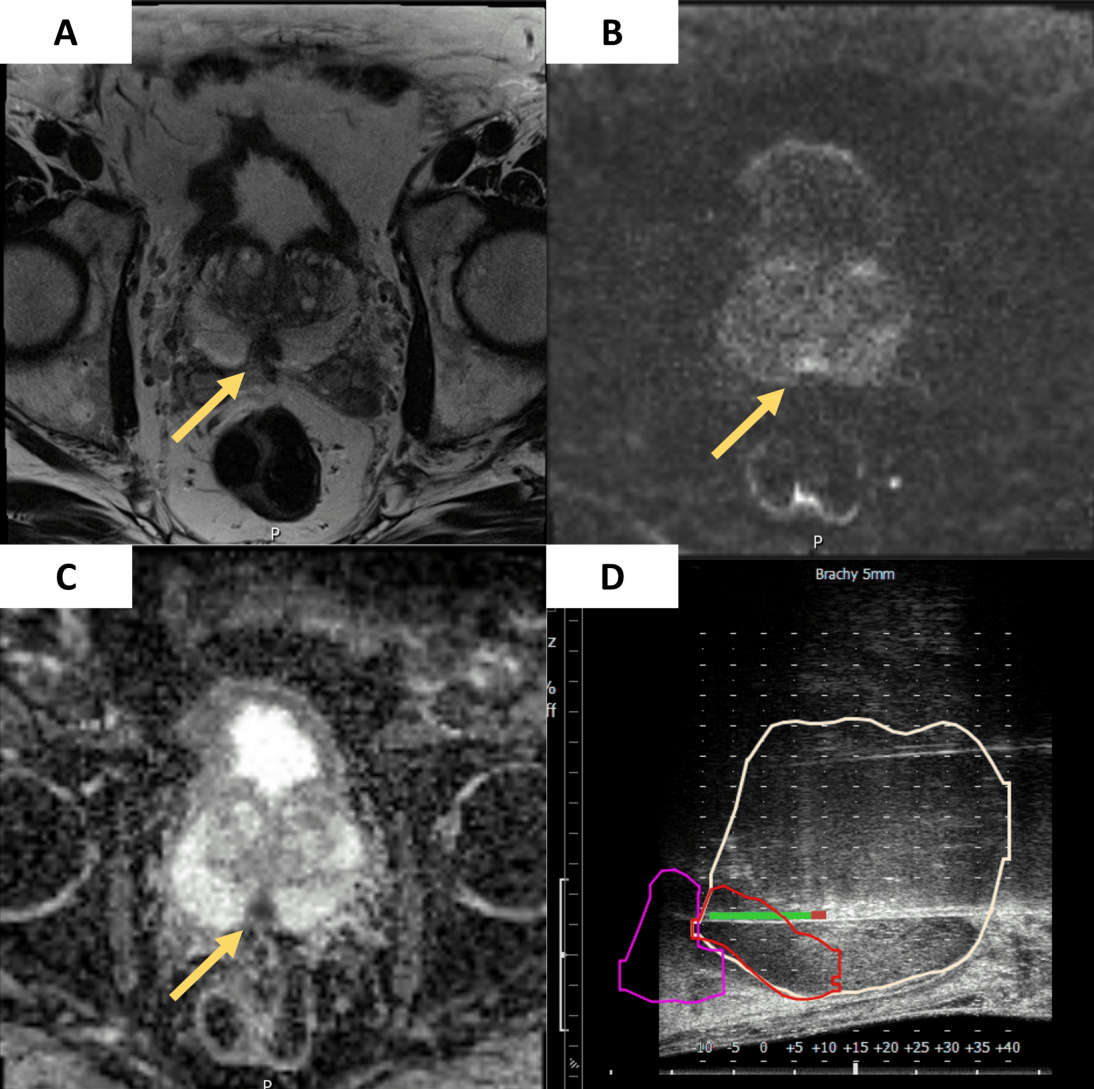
(A) Axial T2-weighted image with a nodular hypointense focal lesion (15 mm) in the peripheric zone (arrow). (B) On DWI with a high b value (1,400), a focal markedly hyperintense lesion (arrow). (C) With a markedly hypointense (arrow) value on the ADC map, consistent with a PI-RADS 5 lesion. (D) sagittal ultrasound view of the fusion-guided prostate biopsy, showing the prostate gland with the highlighted targeted lesion ADC: apparent diffusion coefficient, PI-RADS: Prostate Imaging Reporting and Data System, DWI: diffusion-weighted imaging
